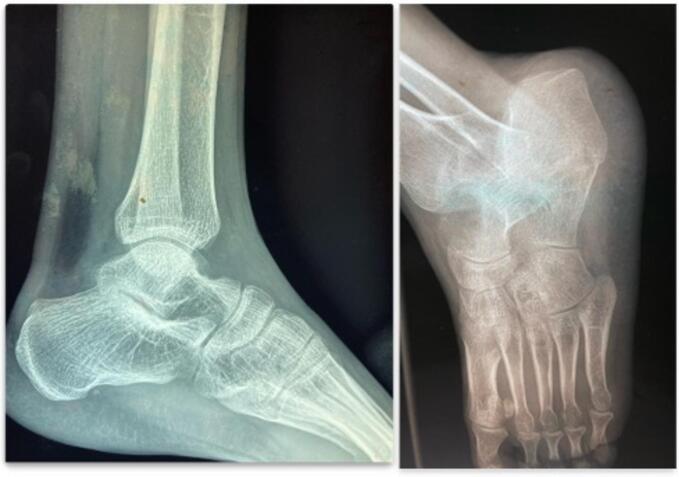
Lateral and 3/4 X-ray of the left ankle.
